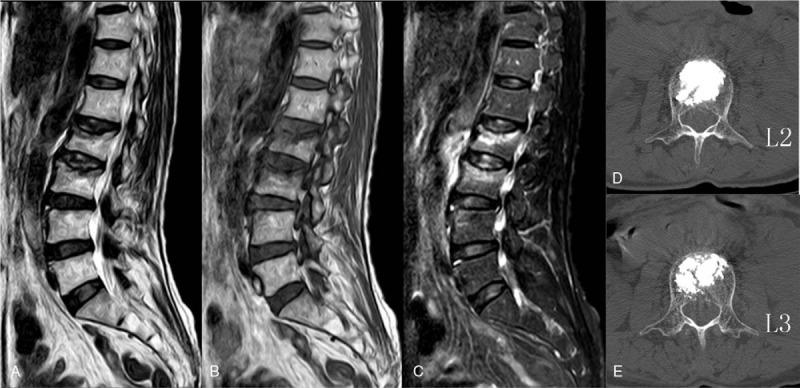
A 62-year-old woman suffered from severe low back pain after an accidental fall 10 d ago. The preoperative MRI (A, B, C) showed osteoporotic vertebral compression fracture of L2 and L3. Postoperative CT (D, E) showed the satisfaction of bone cement was satisfactory. CT = computer tomography, MRI = magnetic resonance imaging.
